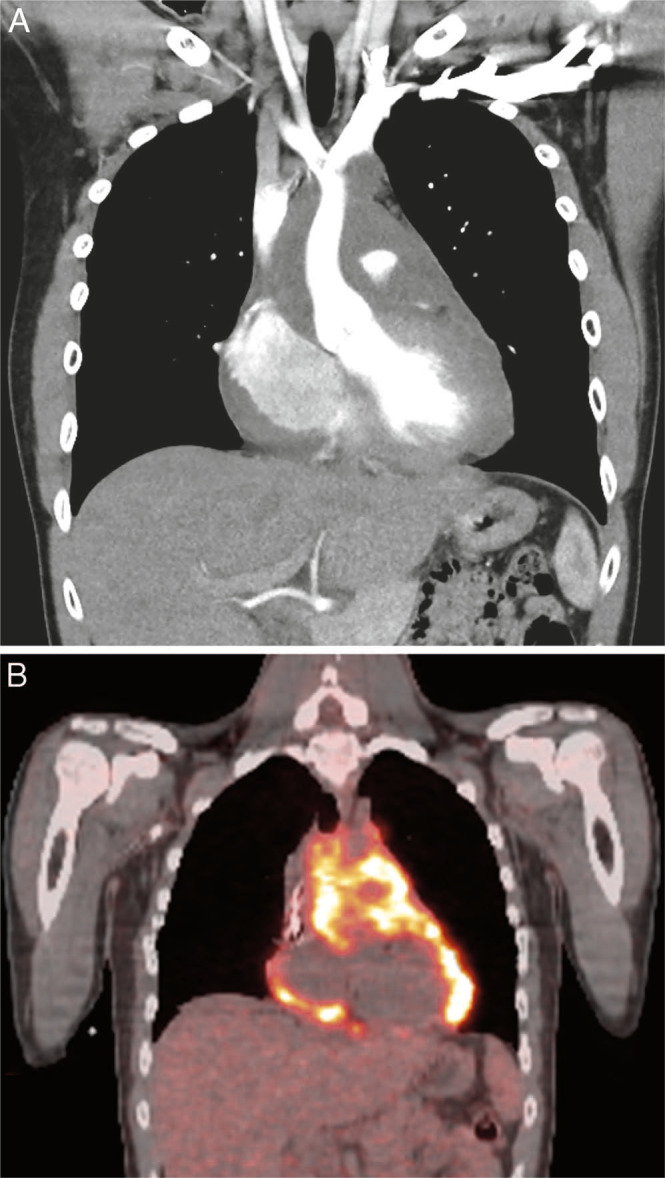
Coronal view of computed tomography scan (A) and positron emission tomography (PET) scan (B) demonstrating extensive sarcoma involving the mediastinum and pericardium.
